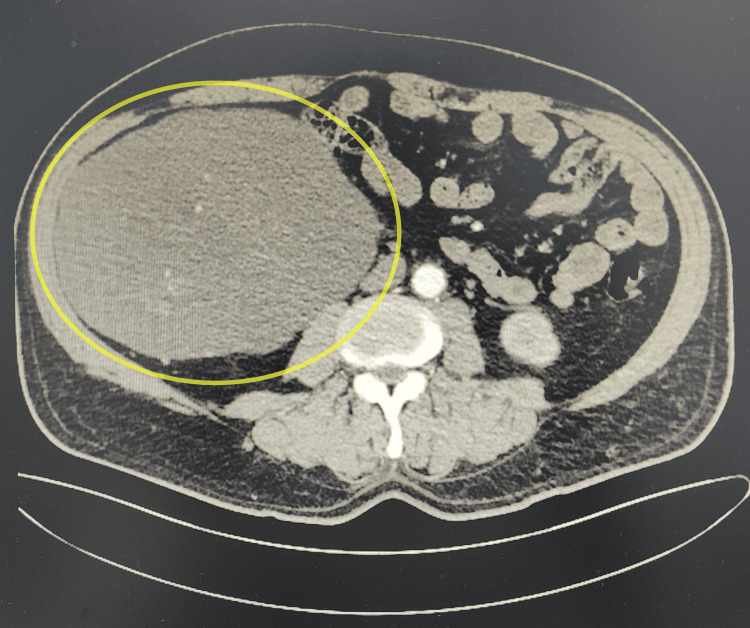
Axial CT scan of the abdomen The yellow circle indicates the retroperitoneal mass.
